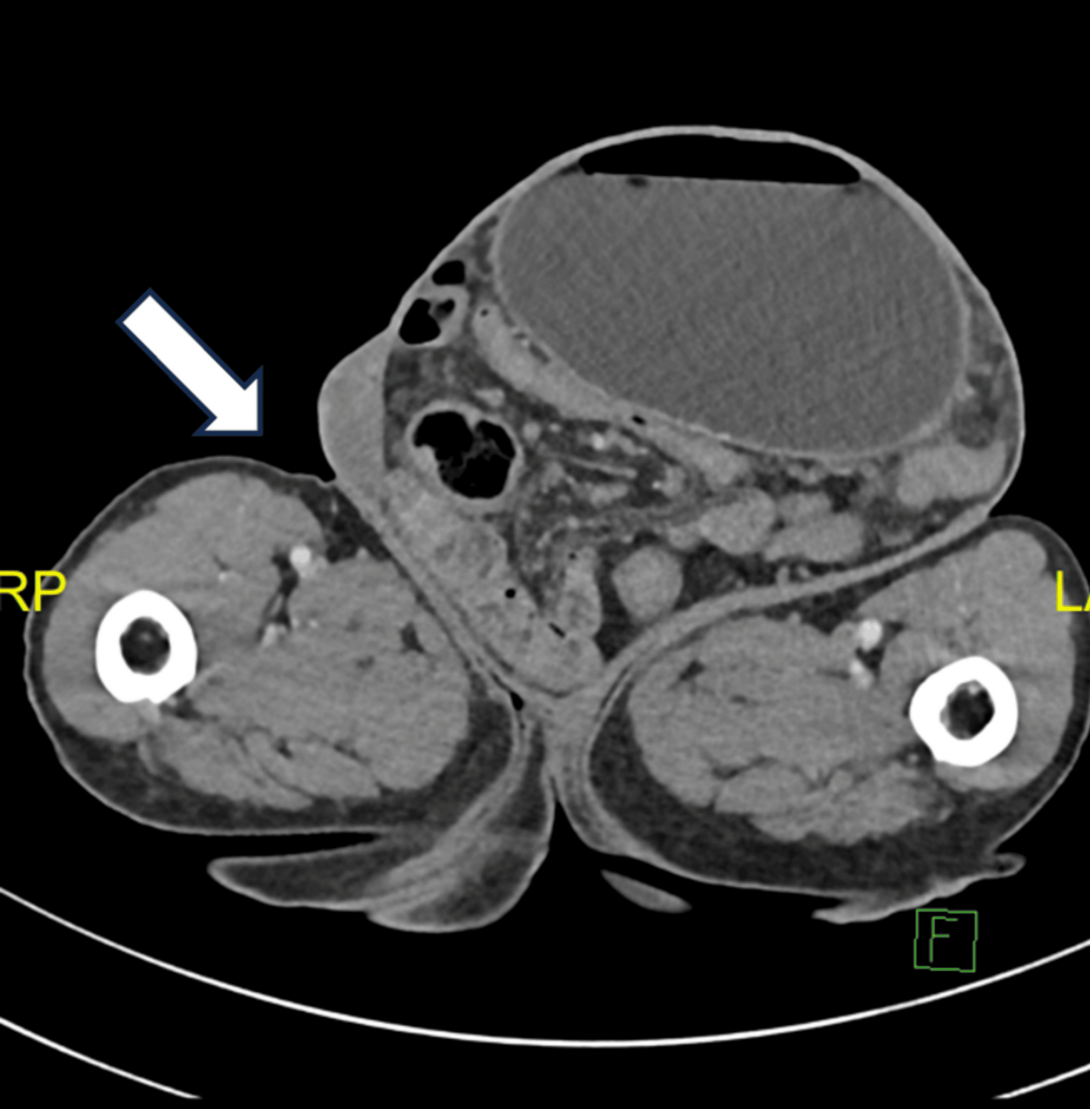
Computed tomography of the abdomen and pelvis showing dilated stomach within the left inguinal hernia sac.
